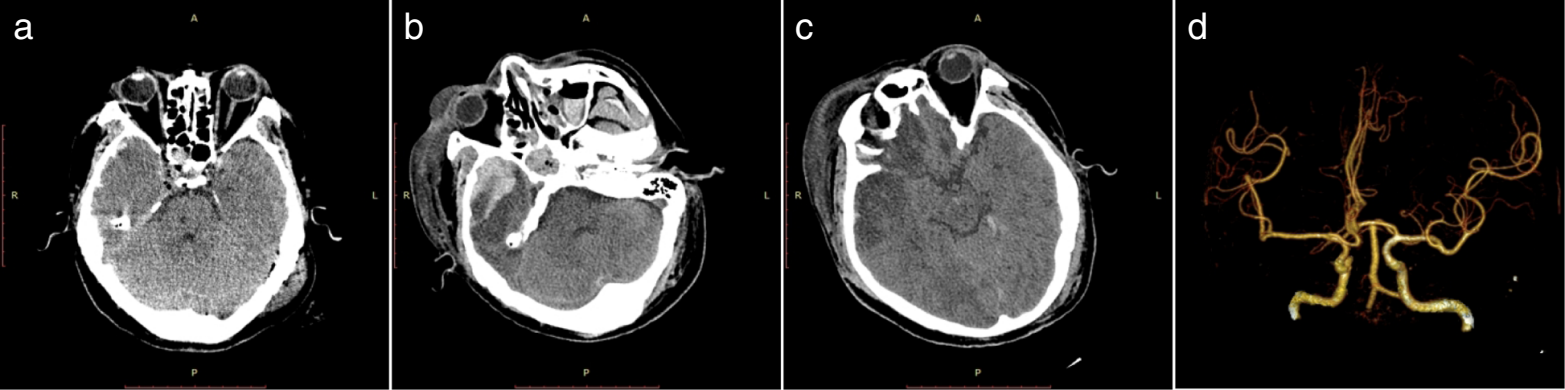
Posttraumatic brain computed tomography (CT) scan. (a) Brain CT scan on admission. (b) Brain CT scan on the second day after admission revealed a larger traumatic intracerebral hemorrhage. (c) Brain CT scan on the second day after admission revealed posttraumatic cerebral infarction. (d) Brain CT angiography
